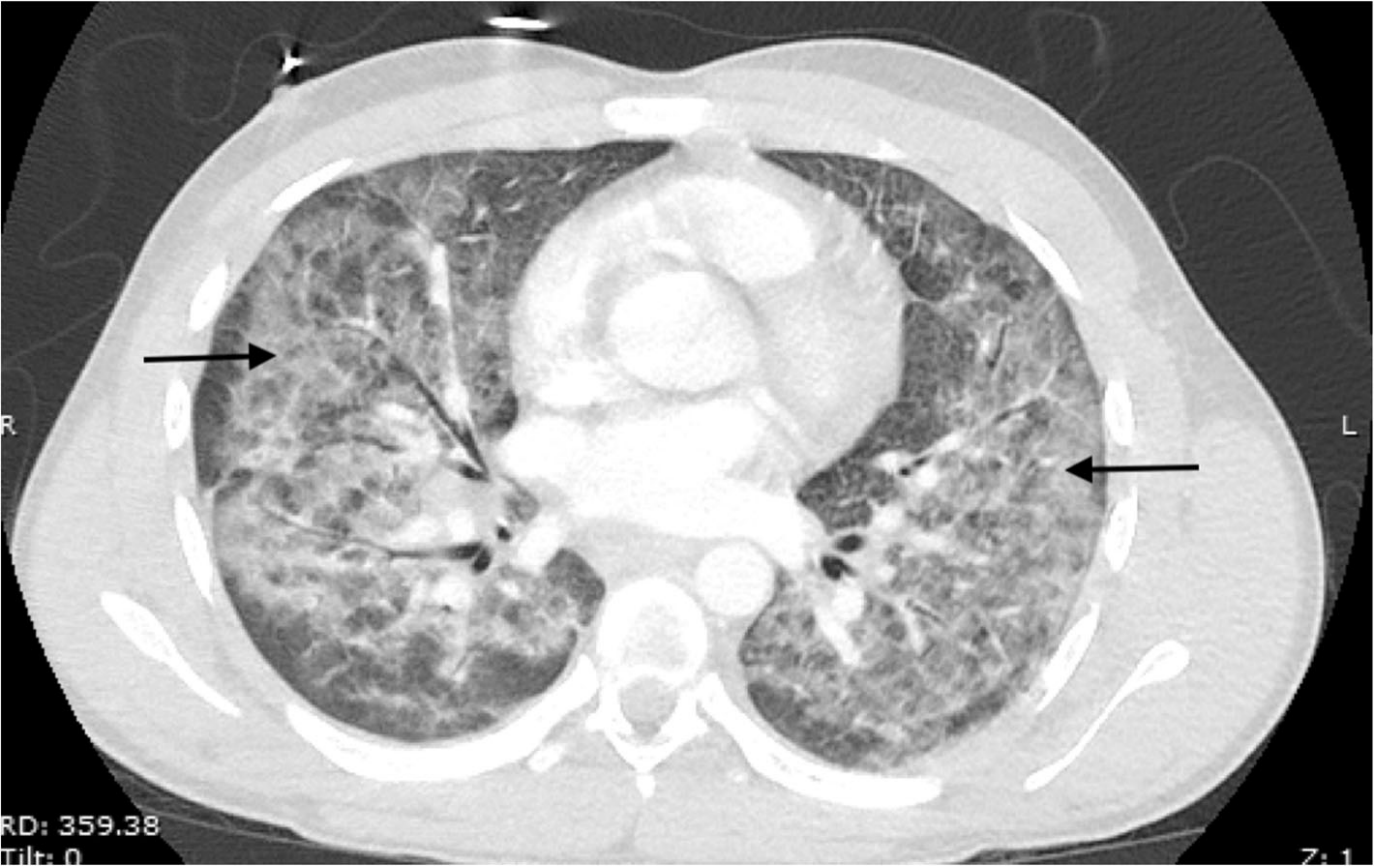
Axial view computed tomographic scan of the chest demonstrating diffuse central predominant interstitial opacities (*black arrows*)
